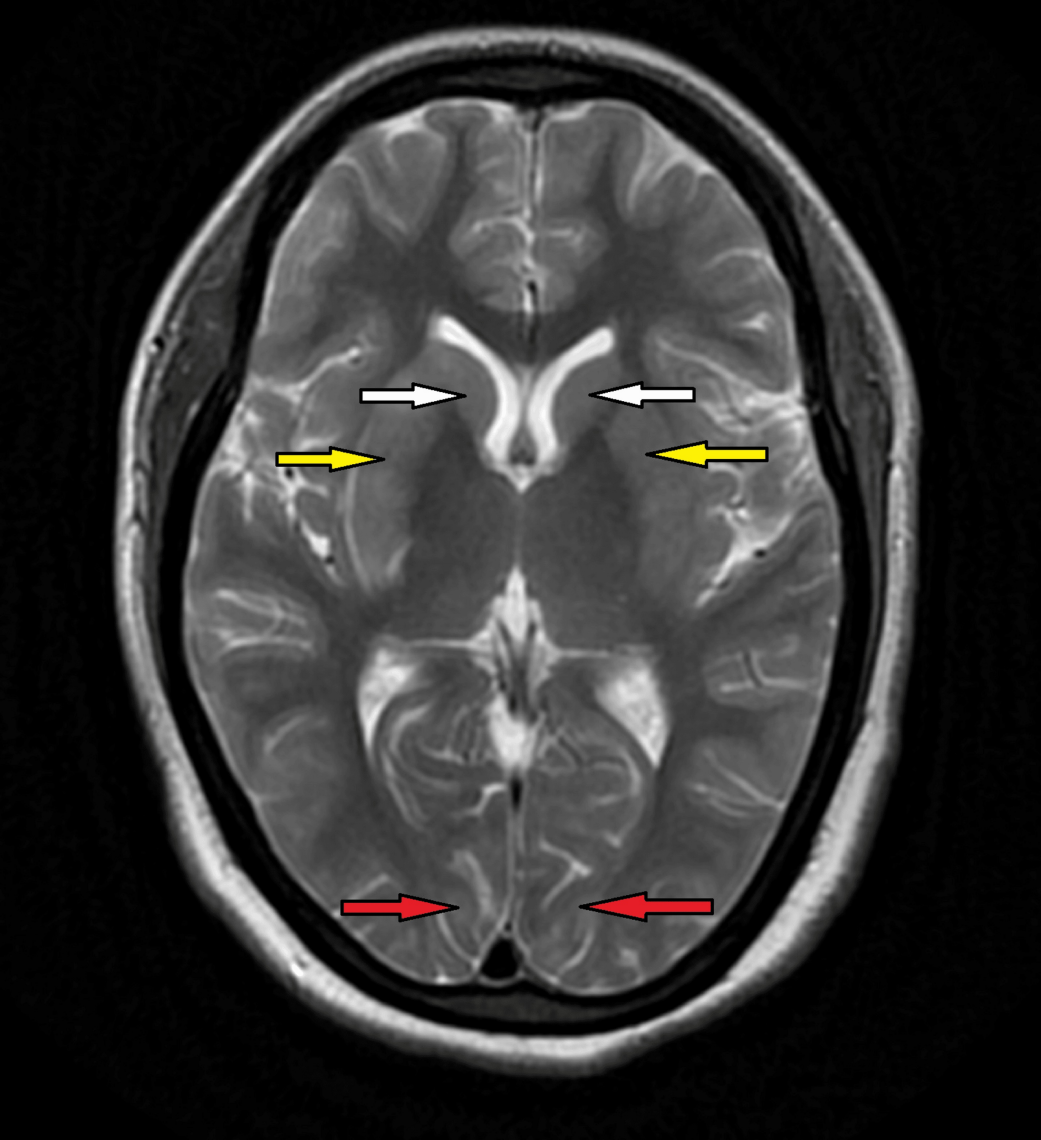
MRI brain (T2W) showing bilateral hyperintense lesions at multiple sites. White arrows show hyperintensities in the caudate nucleus bilaterally; Yellow arrows show hyperintensities in the putamen region bilaterally; Red arrows show hyperintensities in the occipital region bilaterally. MRI: magnetic resonance imaging; T2W: T2 weighted image
